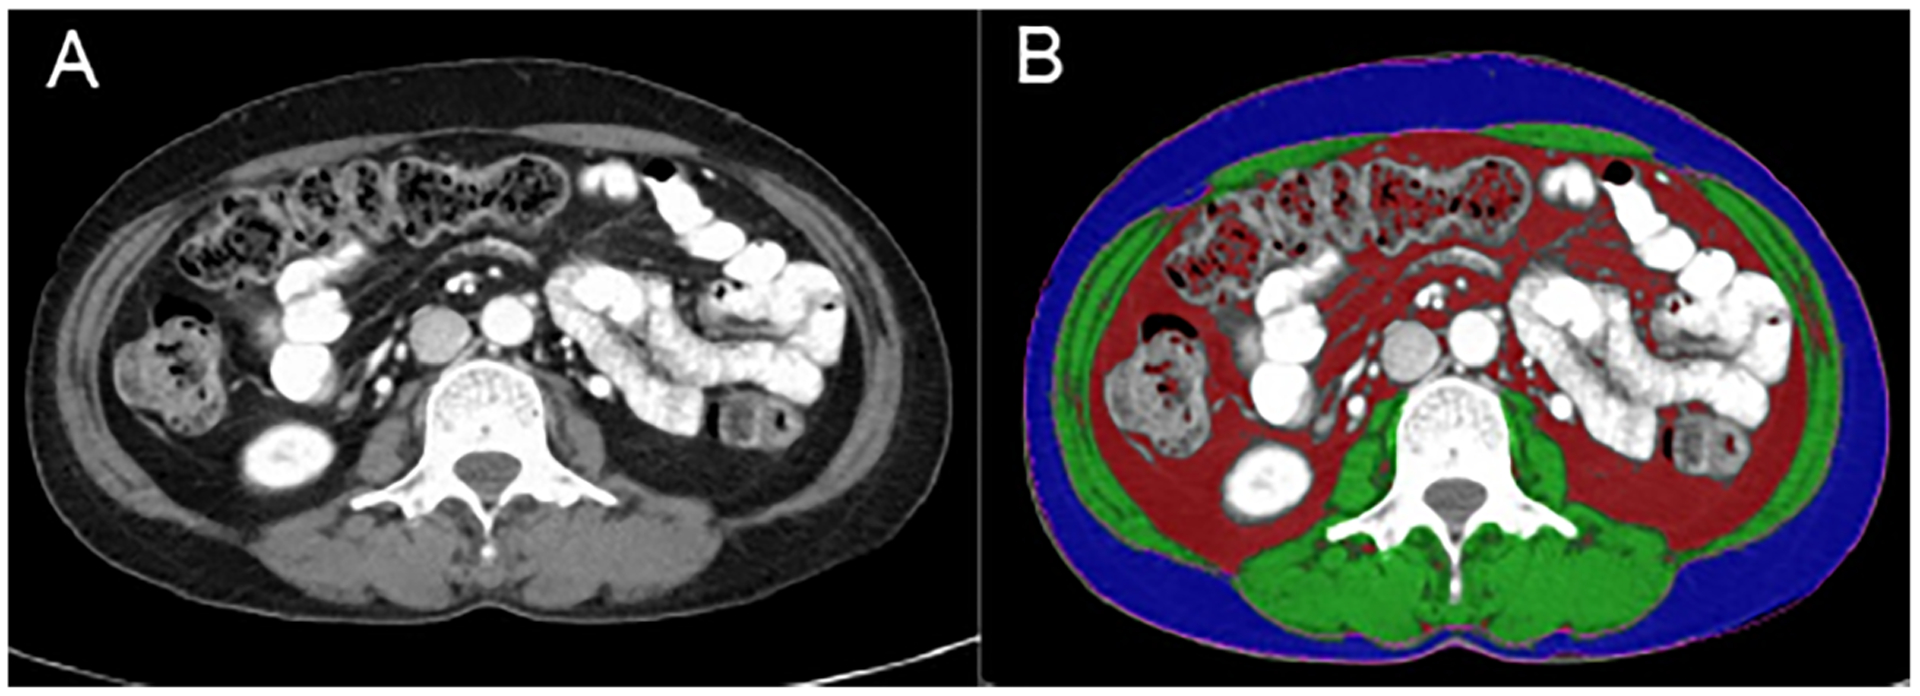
Semi-automated body composition analysis. (A) Example of an axial computed tomography slice at the level of L3. (B) Segmentation of body composition using iNtuition software. Green = skeletal muscle, blue = subcutaneous adipose tissue, red = visceral adipose tissue.
